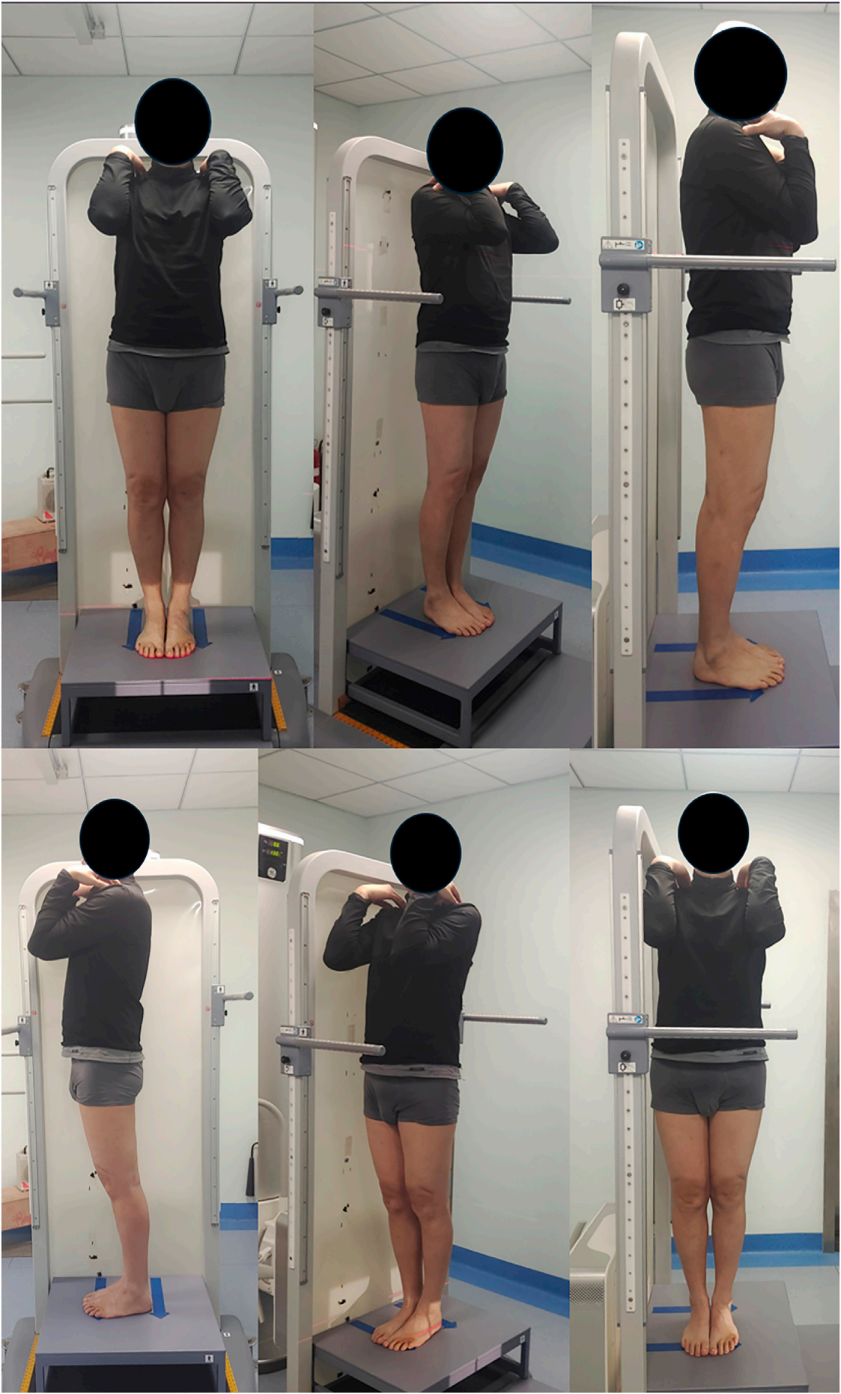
Full length lower limb X-ray in standard standing position in different perspective: The patient stood with his back against the camera bed and was upright facing the ball tube. Put his hands on the shoulders, and the inside of the calcaneus and the inside of the first metatarsal bone were attached to each other, the patella and toe were facing the front, and the back edge was close to the camera bed.
